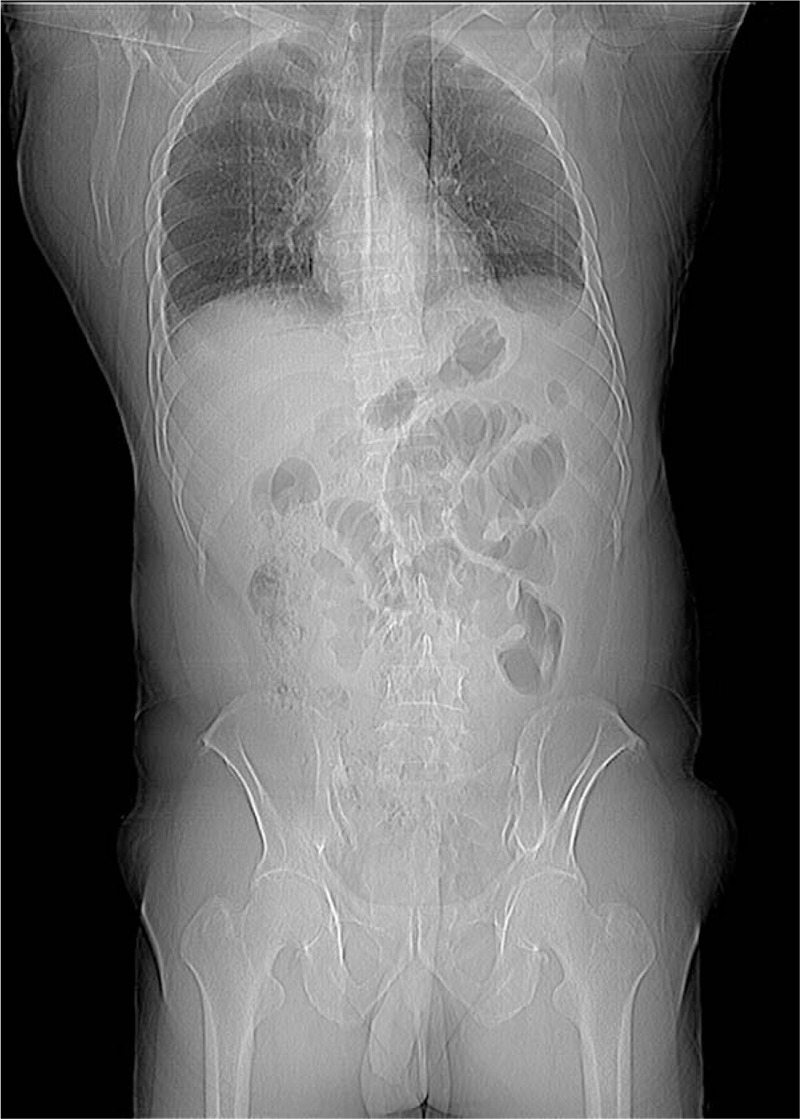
Multiplanar coronal reconstruction computed tomography images showed cauliflower-like appearance of the abdomen and small intestine.
